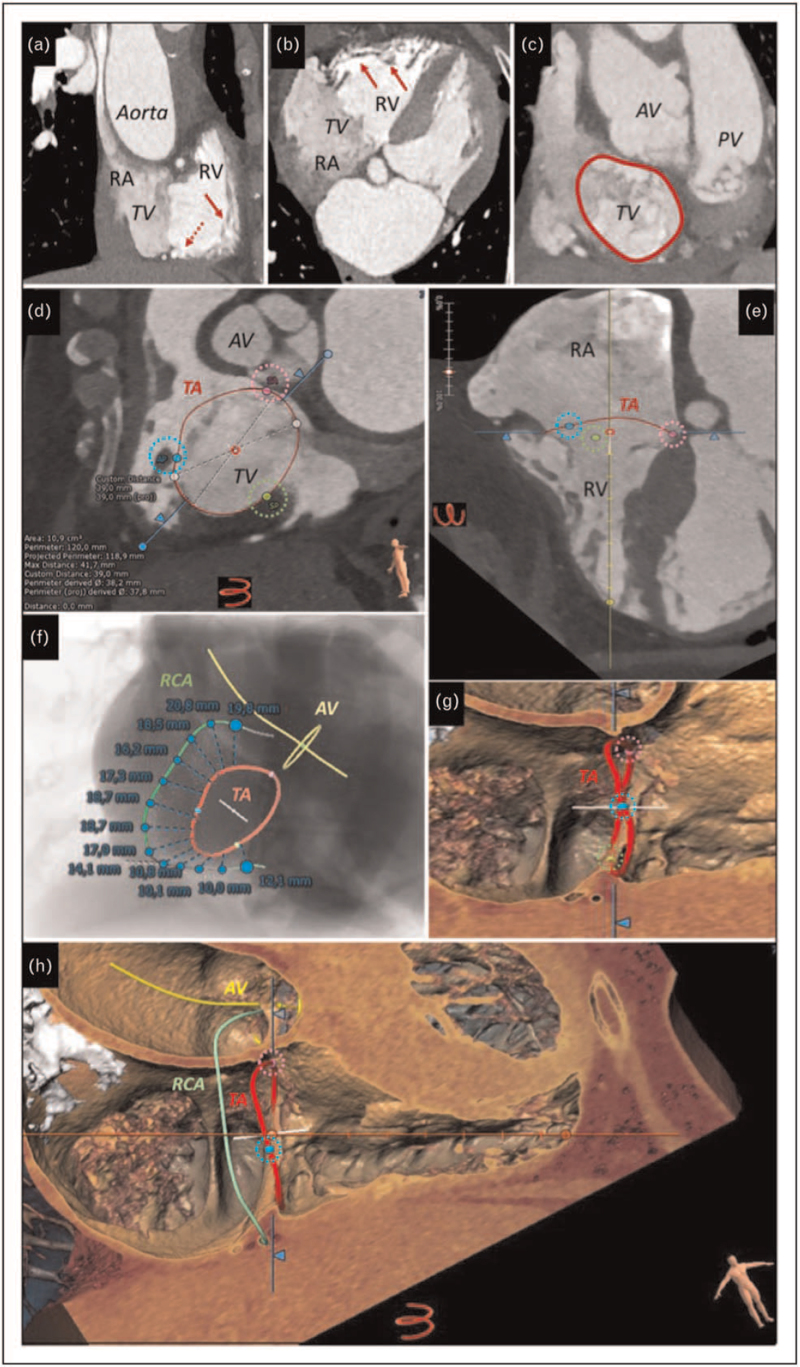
Cardiac computed tomography assessment of the tricuspid valve, right ventricle, chordae (dashed red arrow) and subvalvular apparatus (red arrows), using an inflow-outflow right ventricular view (a) and a 4-chamber view (b). Tomographic reconstruction of the tricuspid annulus with measurement of annular perimeter (c; courtesy of Dr Giuseppe Muscogiuri, Centro Cardiologico Monzino IRCCS, Milan, Italy). Dedicated software analysis providing three-dimensional reconstruction of the tricuspid annulus (red) and measurement of circumference, area and diameters using cardiac computed tomography datasets (d, e; courtesy of Pie Medical Imaging, The Netherlands); identification of commissures is provided by the software (septal-anterior commissure, dashed pink circle; antero-posterior commissure, light blue dashed circle; septal-posterior commissure, green dashed circle). Localization of surrounding structure by dedicated tricuspid valve software using computed tomography derived virtual angio, providing localization of the aortic valve and identification of the right coronary artery; distance between the tricuspid annulus and the right coronary artery is automatically measured by the software (f; courtesy of Pie Medical Imaging, The Netherlands). A zoomed three-dimensional cardiac computed tomography display of the right atrio-ventricular junction showing the three-dimensional reconstruction of the tricuspid annulus and identification of commissures (g; courtesy of Pie Medical Imaging, The Netherlands). Three-dimensional cardiac computed tomography rendering of the right heart with three-dimensional reconstruction of the tricuspid annulus, identification of commissures and anatomical relationship with the aortic valve and right coronary artery (h; courtesy of Pie Medical Imaging, The Netherlands). AV, aortic valve; PV, pulmonary valve; RA, right atrium; RCA, right coronary artery; RV, right ventricle; TA, tricuspid annulus; TV, tricuspid valve.
